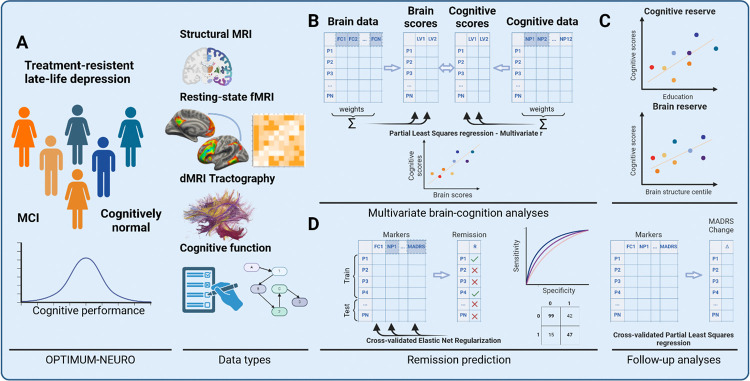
Overview of study design and analyses. We included participants with various levels of cognitive function, MRI and clinical data in our analyses (A). Across all participants, we conducted three multivariate partial least squares (PLS) regressions (B). The first PLS model tested for associations between functional connectivity (FC) and cognitive function; the second PLS model tested for associations between fractional anisotropy, a measure of white matter tract integrity, and cognitive function; the third PLS tested for associations between gray matter and cognitive function. A total of 6 cognitive domains were included. We followed up the PLS analyses by testing for associations between PLS brain and cognitive scores with education and brain structural reserve (C). In addition, in a separate set of analyses we used cross-validated logistic regression models to predict patients’ remission status using clinical, demographic, cognitive and neuroimaging markers (D). Area-under-the-curve and confusion matrices were used to assess and visualize model performance. The time at which ‘baseline’ neuropsychological and MRI assessments were completed relative to the parent OPTIMUM trial is shown in the bottom panel, with more details available in Supplementary Section S2. Abbreviations: OPTIMUM-NEURO: Optimizing Outcomes of Treatment-Resistant Depression in Older Adults; MCI: Mild Cognitive Impairment; Montgomery-Asberg Depression Rating Scale; LV: latent variable; P1-PN: participants 1:N; NP: neuropsychology and MRI assessment
